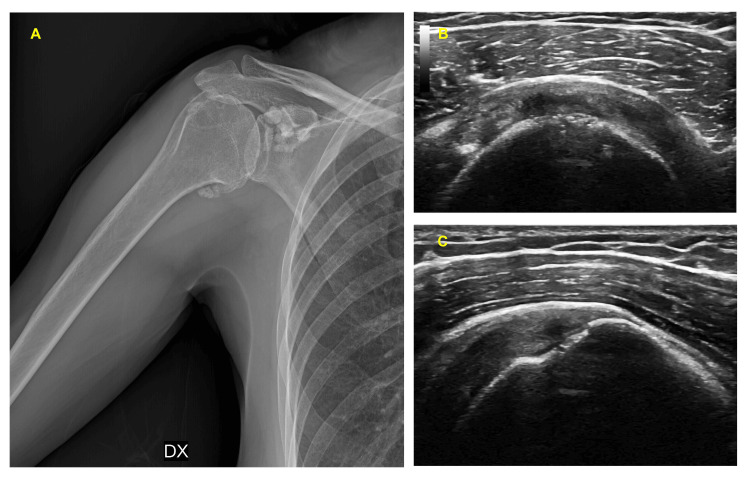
Patient 5. (A) X-ray of the right shoulder of patient 5 with glenohumeral osteoarthritis with gross osteophyte of the lower margin and sub-coracoid and soft tissue peri-diaphyseal calcification of soft tissue. (B-C) Ultrasound of supraspinatus tendon with an intrasubstance partial tear.
